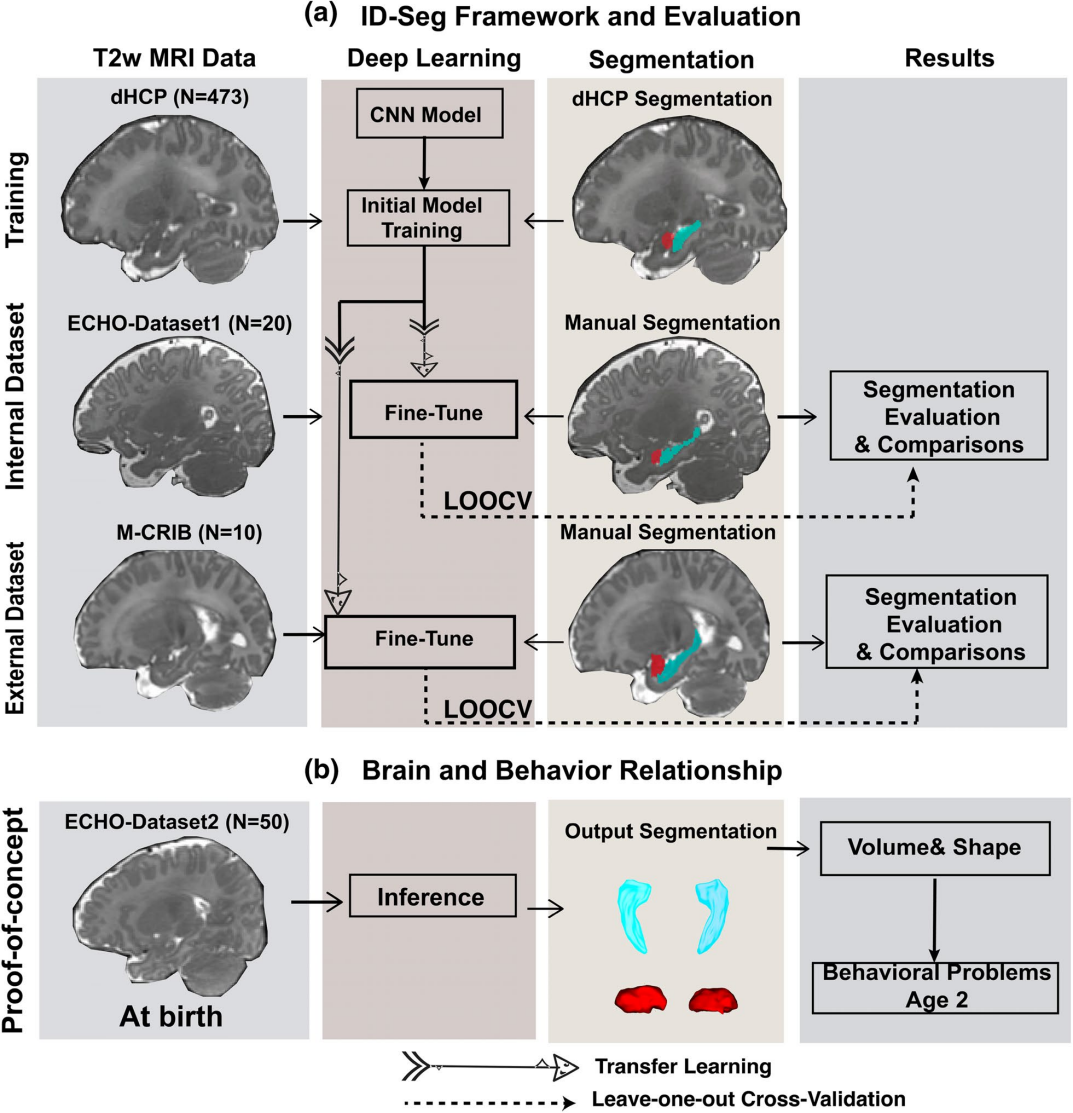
Overview of this study. a Using 3 independent infant MRI datasets through a transfer-learning approach, we trained, fine-tuned, and cross-validated a deep-learning segmentation framework (ID-Seg) for hippocampus and amygdala, both with internal and external datasets; b we further explored the prospective associations between morphometric measures (left and right hippocampus and amygdala) in infants and behavior problems at age 2. *Cyan color represents segmented hippocampus, and red represents segmented amygdala. *LOOCV* leave-one-out cross-validation
